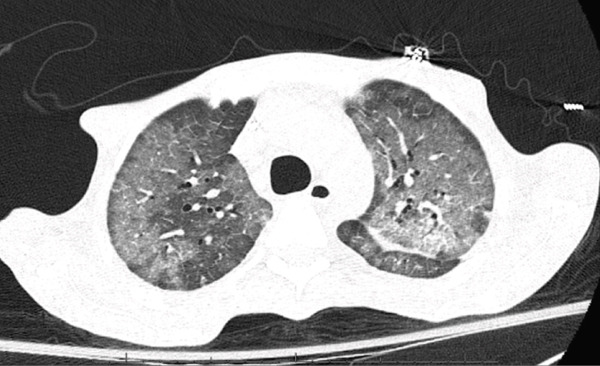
CT chest demonstrating diffuse bilateral ground‐glass opacities with confluent consolidations in the lower lobes and extensive mediastinal lymphadenopathy.
